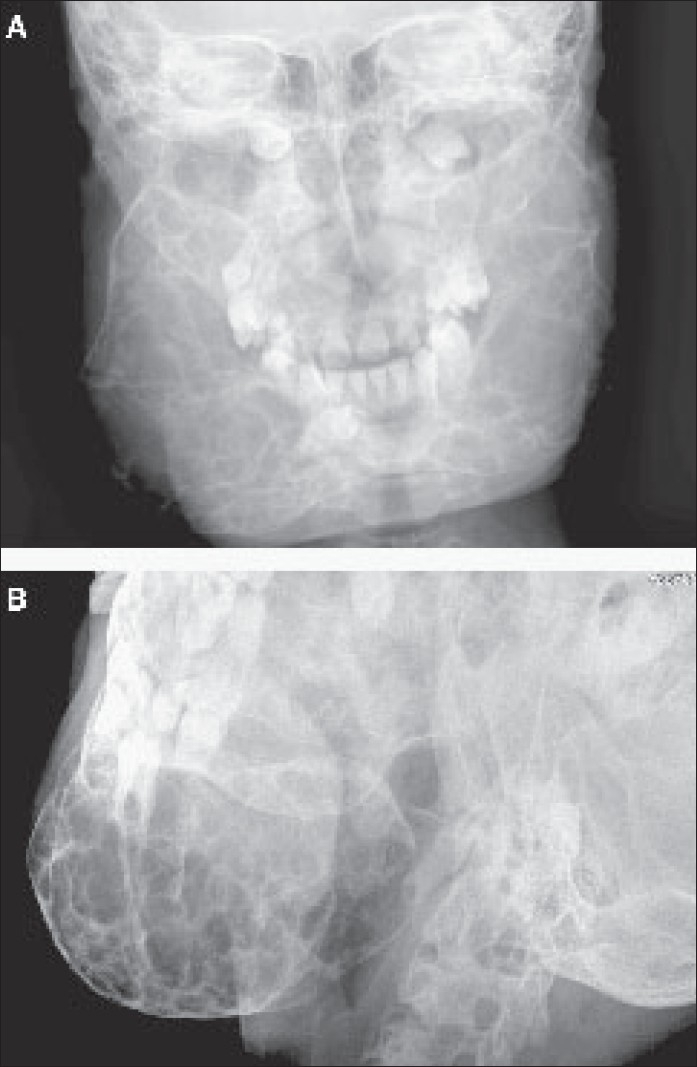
Frontal (A) and oblique (B) radiographs show expansile remodeling of the maxilla and mandible with multilocular radiolucent lesions causing cortical thinning
